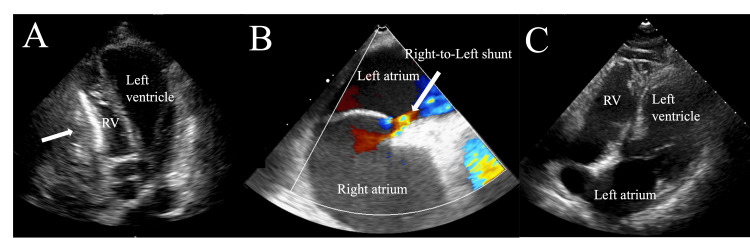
Echocardiographic evidence of right heart compression and intracardiac shunting (A) TTE, apical four-chamber view, showing the compression of the RV due to a pericardial hematoma (white arrow). (B) Intraoperative transesophageal echocardiogram with color Doppler showing right-to-left shunting through the patent foramen ovale with turbulent flow and a mosaic color pattern (white arrow). (C) Follow-up TTE apical four-chamber view on hospital day 82 showing the resolution of right-sided heart compression after the surgical removal of the hematoma. TTE: transthoracic echocardiogram; RV: right ventricle
